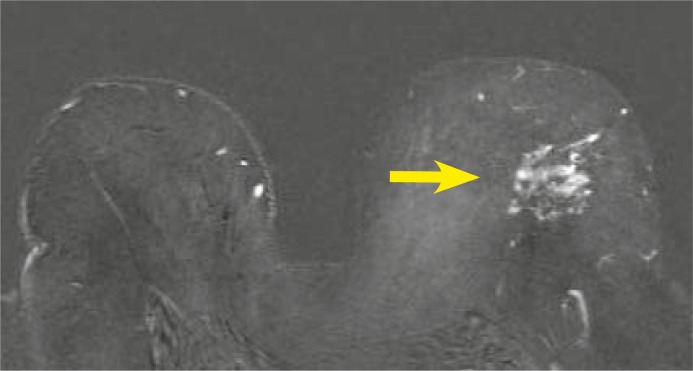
64-year old woman with history of invasive breast carcinoma in her right breast 10 years ago. Mammography and ultrasound images showed discrete architectural distortion in the left breast. US-guided core biopsy was inconclusive. Axial T1-weighted subtracted image after Gadolinium injection (2^nd^ minute) shows regional, heterogeneous, asymmetric, non-mass like enhancement, fast initial increase, post initial wash-out, intermediate signal intensity on T2-weighted images - BI-RADS 5. Pathologic diagnosis through MRI-guided vacuum-assisted biopsy was DCIS.
